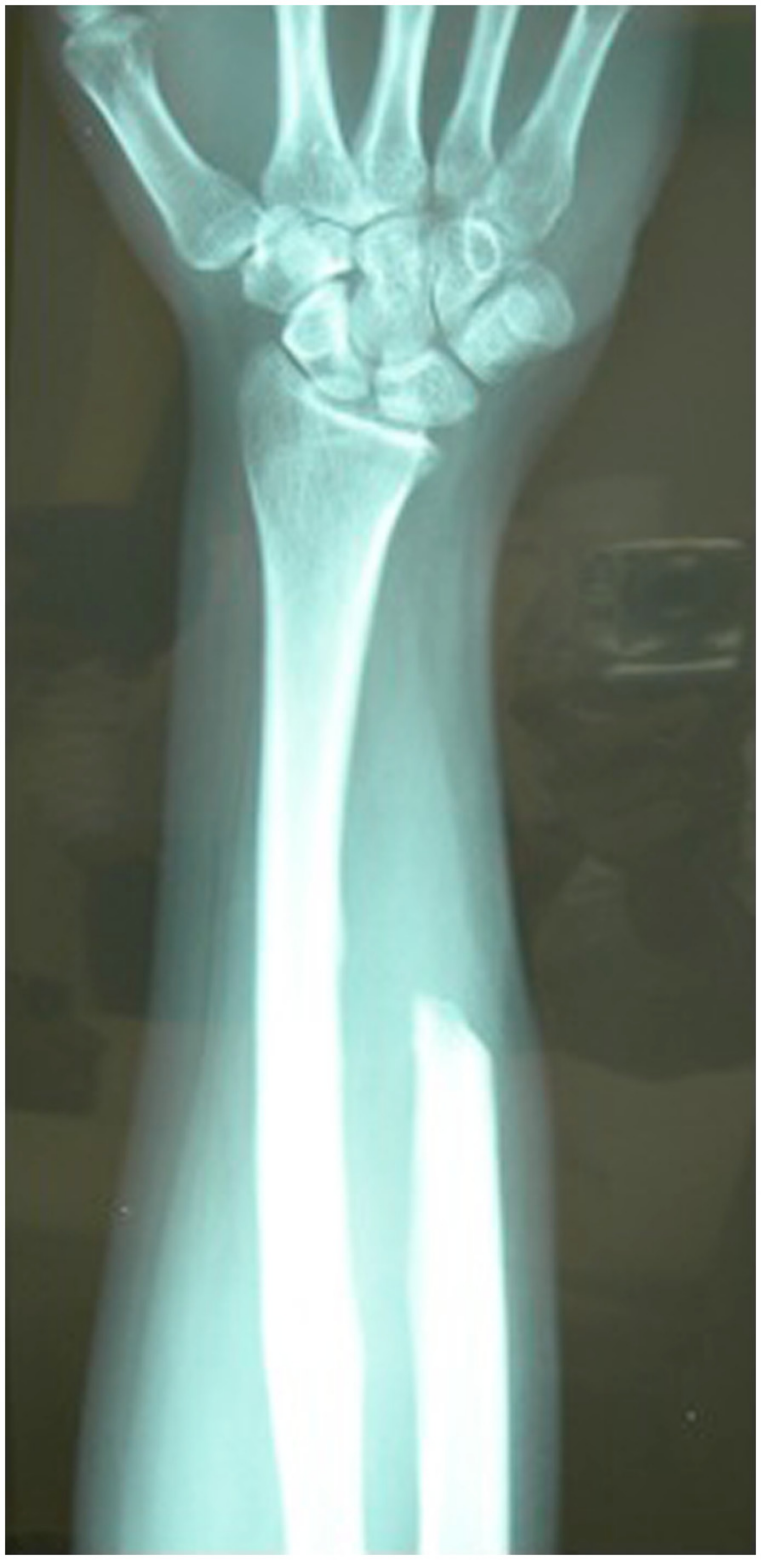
X-rays after distal ulna resection. No mass left in the forearm. The interosseus membrane seems to be free from other ossifying lesions.
